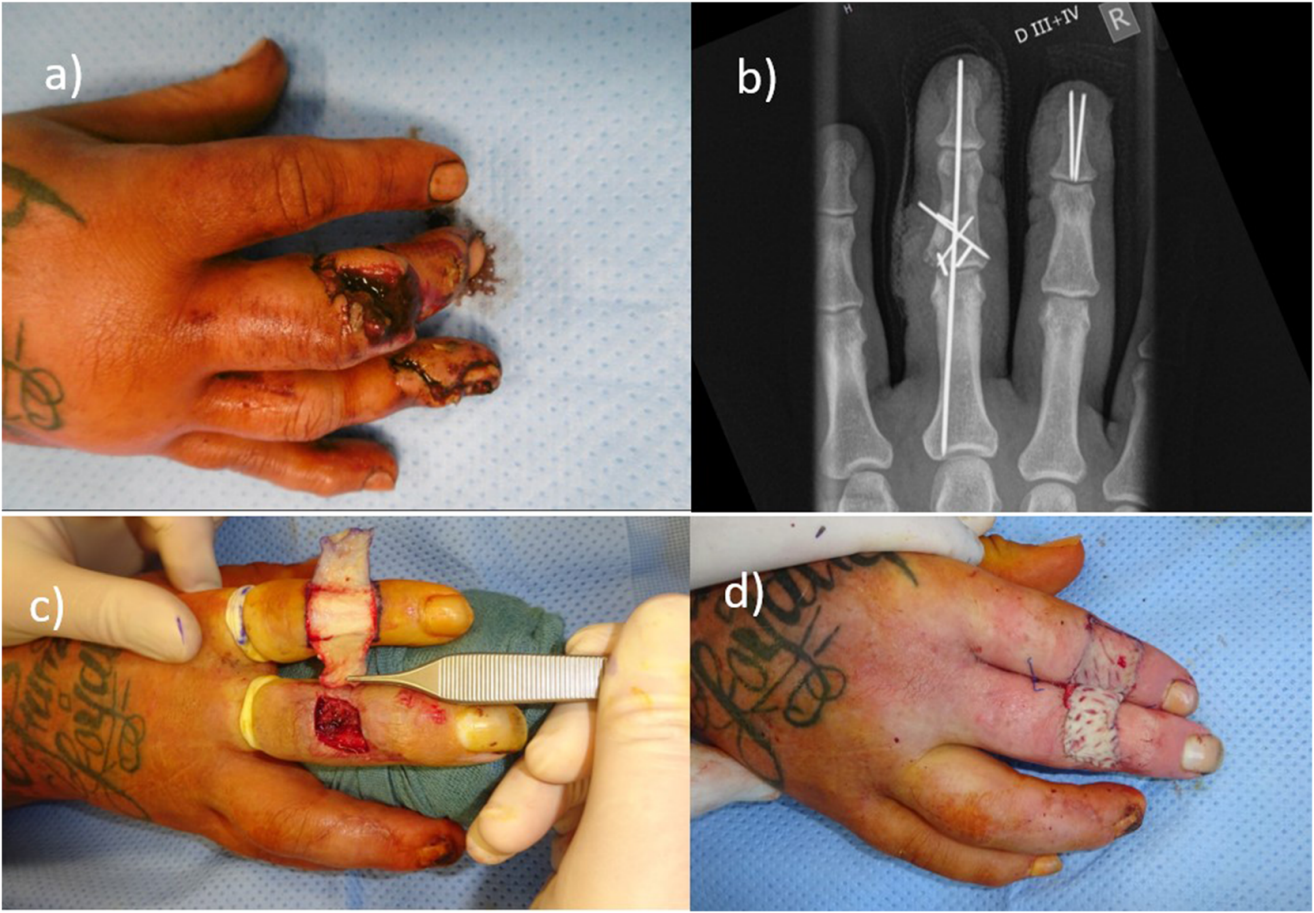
Male patient with saw injury of the right middle and ring finger (a). (b) Postoperative X-ray after K-wire osteosynthesis of the right middle and ring finger. (c)/(d) Soft tissue coverage of the right middle finger with reversed cross-finger flap from the index finger.
